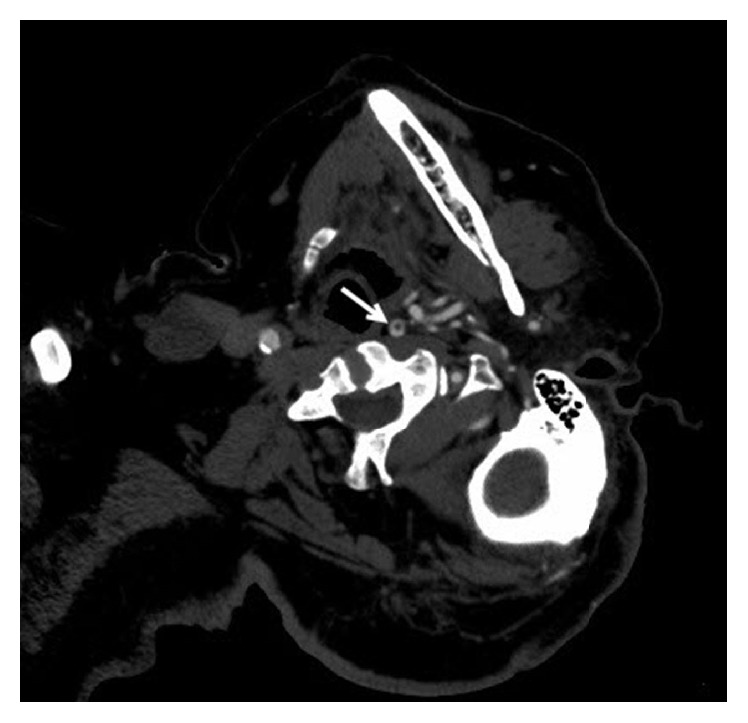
Axial image from CT angiogram demonstrates free floating thrombus within the internal carotid artery lumen “donut sign” (white arrow).
